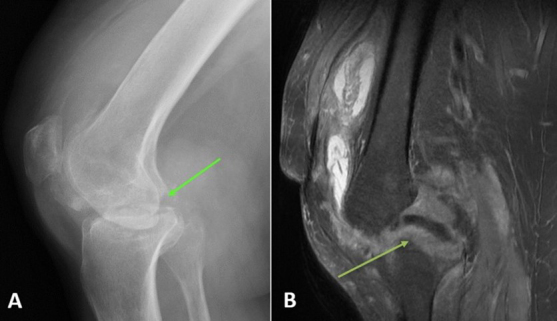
Case 2 status post-irrigation and debridement. (a) Lateral right knee radiograph demonstrating destructive changes in the medial femoral condyle (green arrow) and joint space narrowing. (b) T2-weighted sequence sagittal knee MRI demonstrating the collapse of the lateral tibial plateau (yellow arrow), increased synovial thickening, and further maceration of the menisci and cruciate ligaments.
